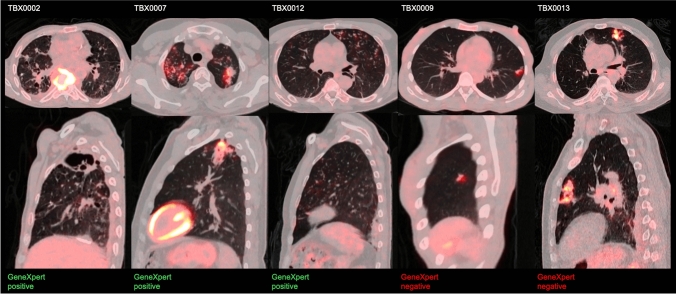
PET/CT imaging of the five patients with increased pulmonary FDG-uptake
